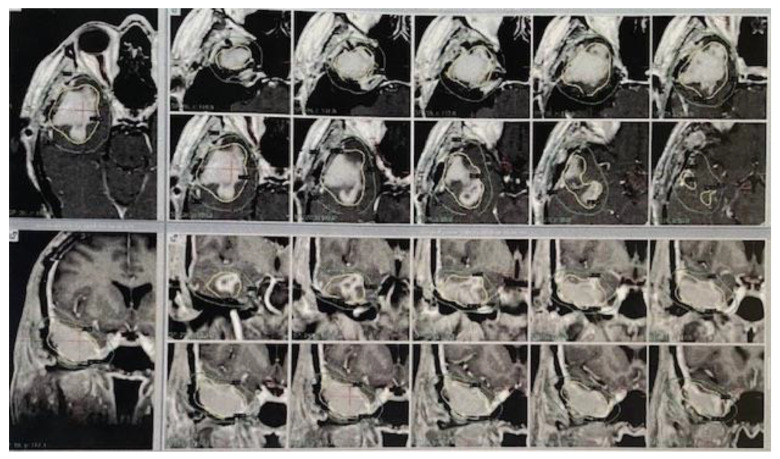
Leskel Gamma plan 10.1.1 Stereotactic MRI T1-weighted with contrast axial (superior lines) and coronal (inferior lines) image views, obtained at 2 mm intervals. The target within yellow lines received maximal dose calculated for this patient (12 Gy-50%), with a total volume of 26.8 cc. All 49 patients received concurrent or adjuvant chemotherapy with GKRS, adhering to the protocol of daily TMZ administered continuously (75 mg per square meter of body surface area daily) during the radiotherapy phase, subsequently succeeded by six cycles of auxiliary TMZ (150 to 200 mg per square meter administered over 5 days within each 28-day cycle). This comprehensive therapeutic approach aimed to optimize the therapeutic outcomes while considering the cognitive concerns associated with conventional radiotherapy.
